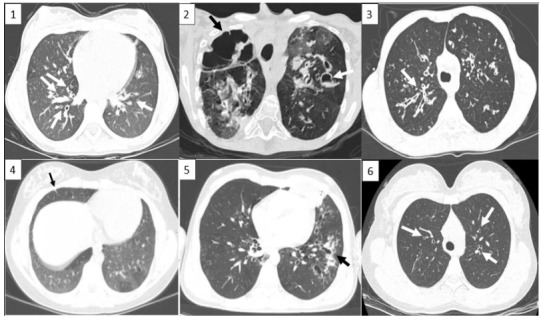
High-resolution computed tomography images of the patients. 1a. Peribonchial thickening, 1b. Cystic areas, 1c. Budding tree sign, 1d. Nonspecific nodule, 1e. Pulmonary infiltration, 1f. Central bronchiectasis.
